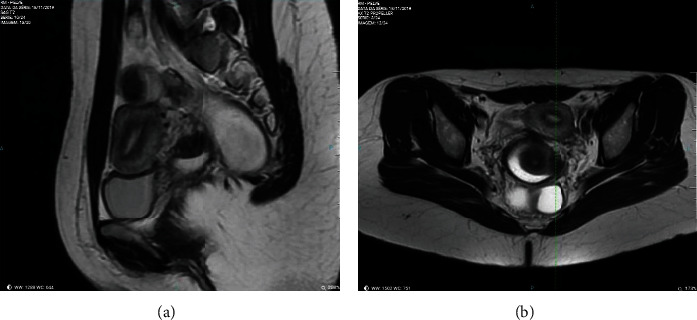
(a) Sagittal T2-weighted MRI. (b) Axial T2-weighted MRI.
